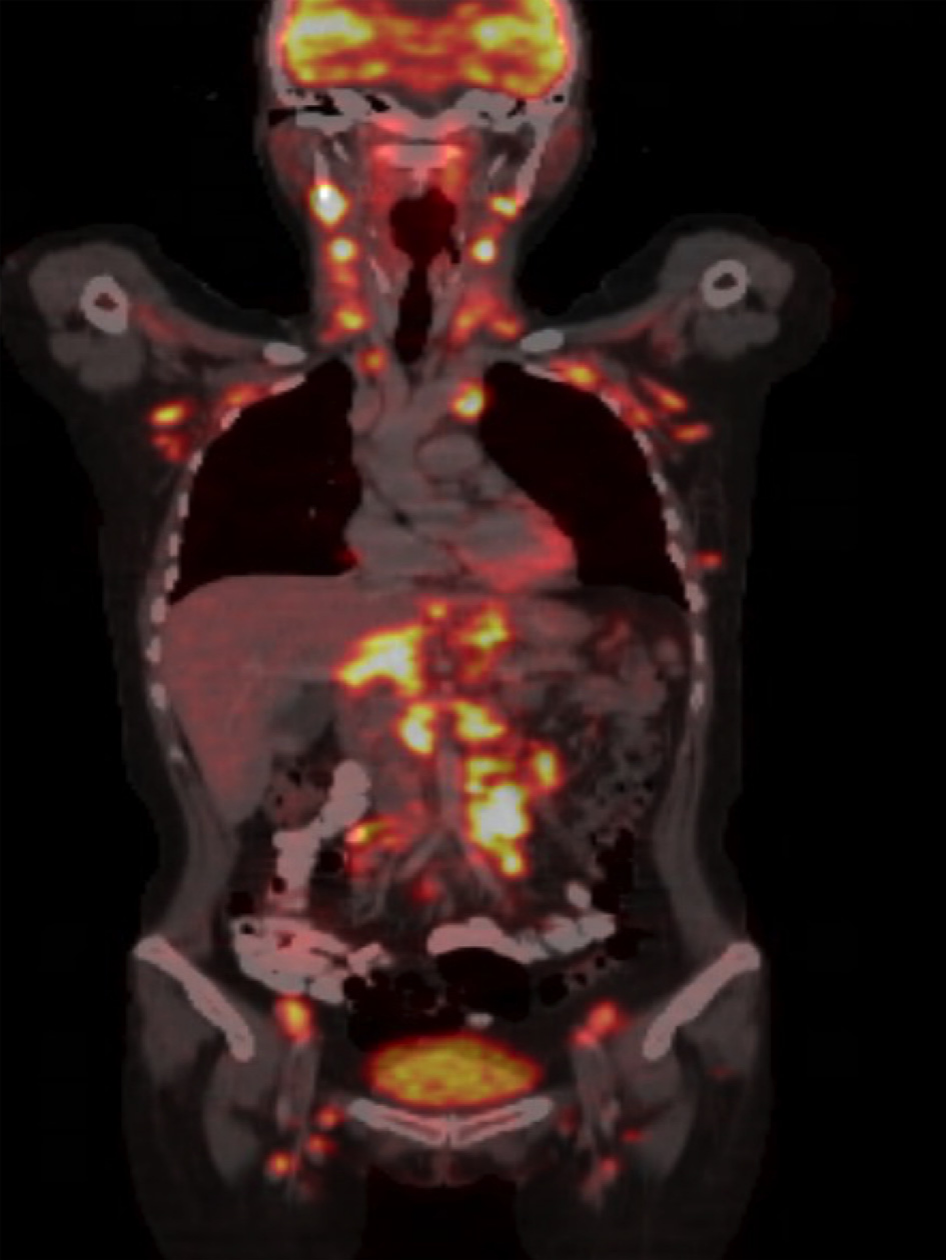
18 F‐FDG PET/CT coronal fusion demonstrating generalized enlargement of cervical, axillary, mediastinal, abdominal, and inguinal lymph nodes, with increased FDG uptake.
